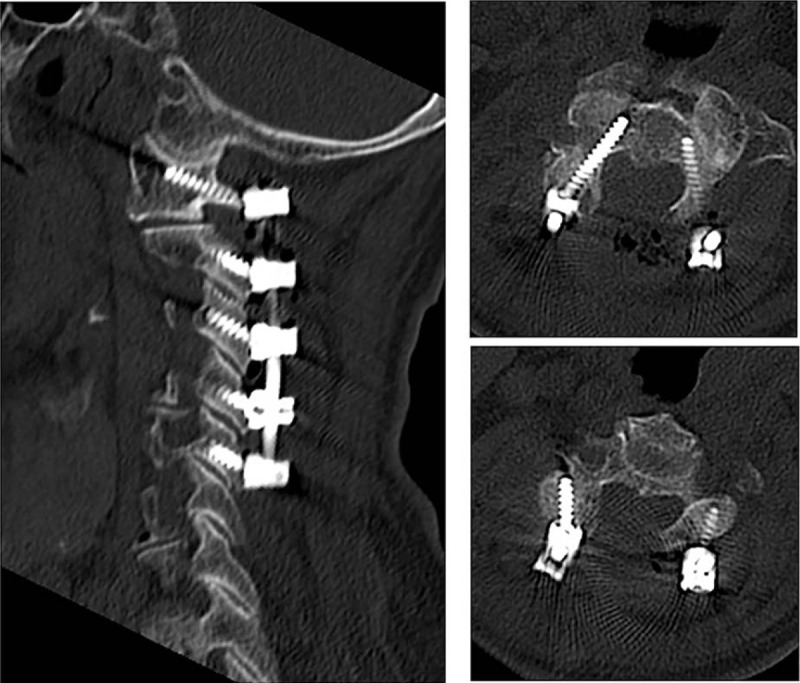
The postoperative computed tomography showed C1-2 vertebral pedicle and C3-5 lateral mass were fixated by posterior screw-rod system. The narrow canal had been corrected.
